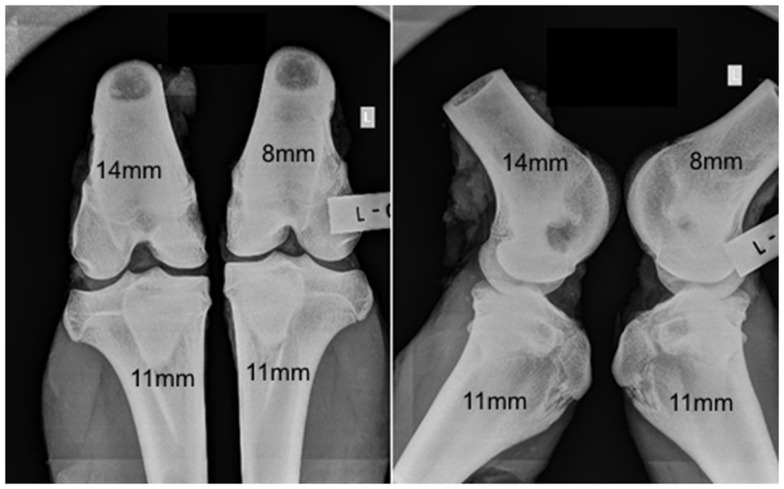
Representative radiographs in anteroposterior (left) and lateral (right) orientations.
